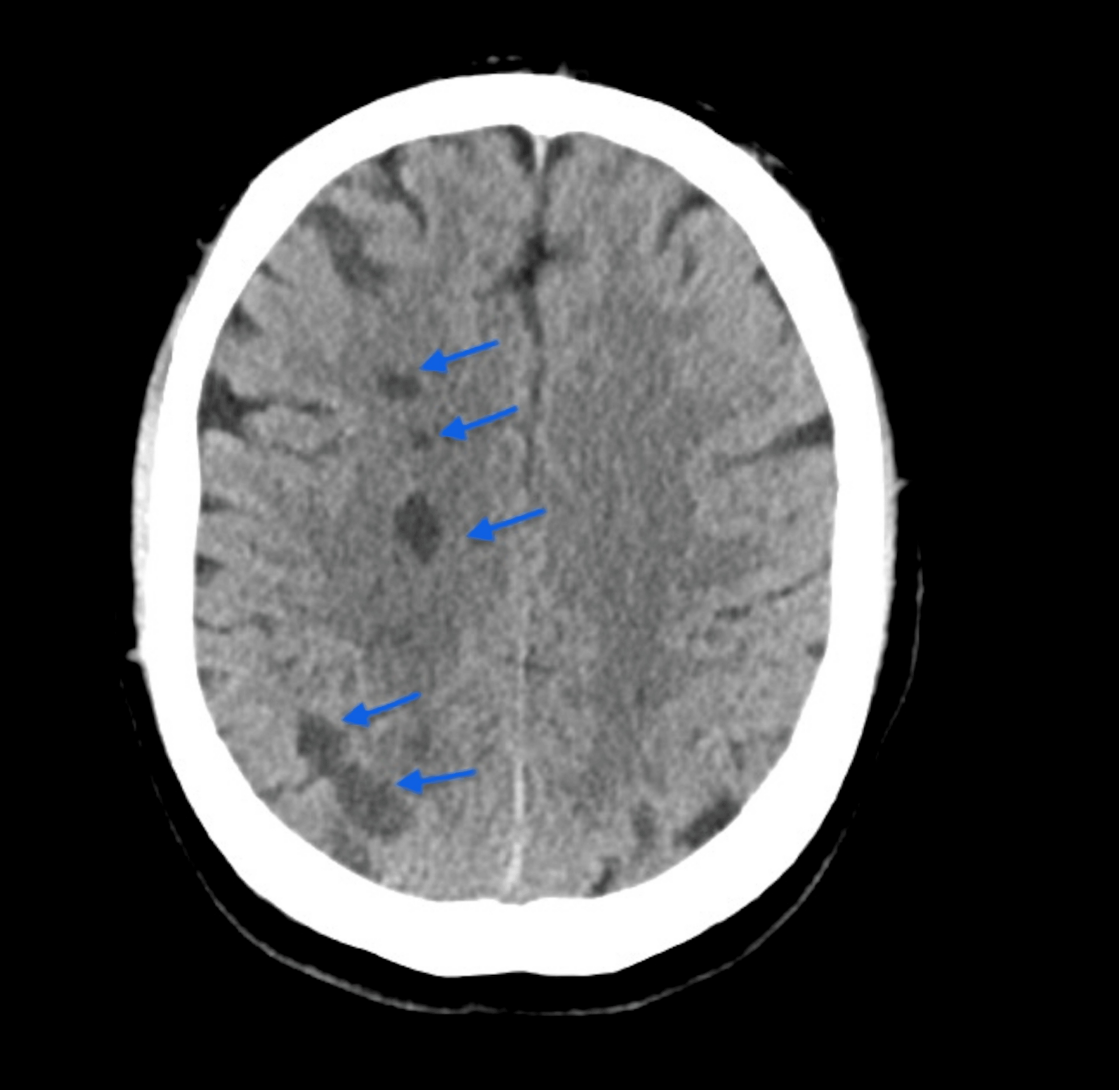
Head CT demonstrating multifocal infarcts within the right cerebral hemisphere (blue arrows)
